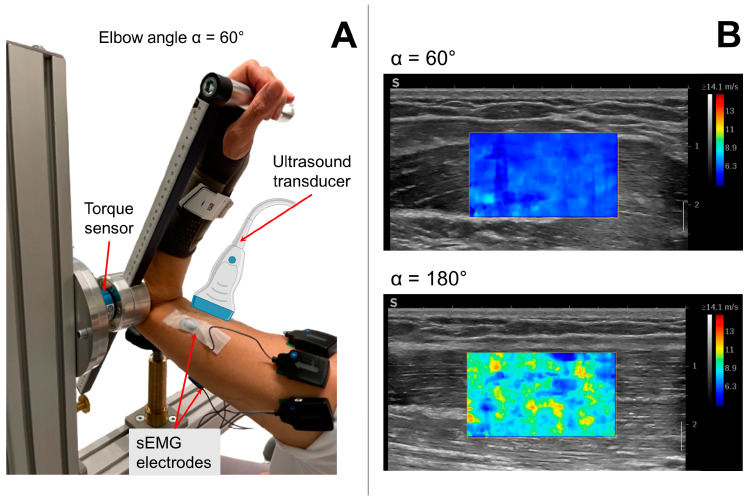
(A) Experimental setup showing a patient’s left arm placed in the custom-made apparatus. The elbow joint axis is aligned with the rotational axis of the torque sensor. Surface electromyography (sEMG) electrodes are positioned at the biceps brachii (BB) and triceps brachii muscle. The ultrasound transducer is placed on top of the BB muscle belly and aligned with muscle fiber direction. (B) Exemplary ultrasound frames including shear wave propagation speed color overlays during 50% isometric ramp contractions for two elbow angles (60°, 180°) studied.
